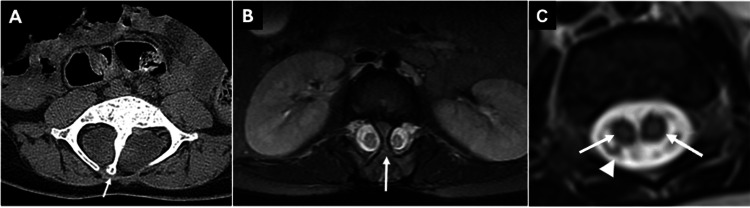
(A) Axial CT image through the lower spine displays findings of a type I diastematomyelia with an osseous septum dividing the spinal canal (arrow) and two separated hemicords. (B) Axial T2 fat-saturated MR image at the level of the kidneys in type I diastematomyelia demonstrating two hemicords separated by an osseous septum (arrow). (C) Axial T2-weighted MR image of the spinal canal shows a single dural sac (arrowhead) containing both hemicords (arrows) in type II diastematomyelia. All images were obtained by the authors from the institutional picture archiving and communication system (PACS). They have been de-identified and used in compliance with institutional policies.
